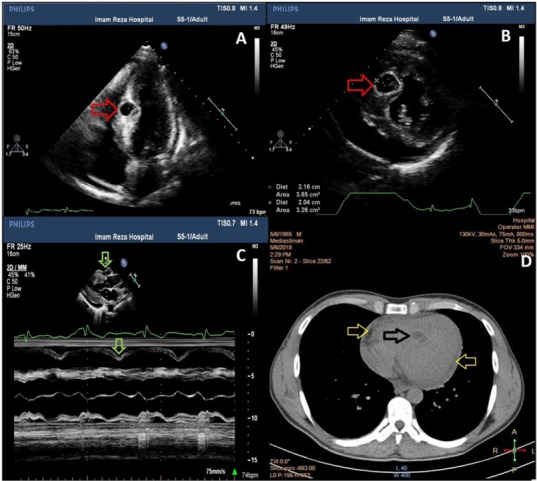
High-resolution computed tomography (HRCT) revealed a hypodense, cystic like structure in IVS and also pericardial effusion (Figure 2D). HRCT of the lungs and CT of abdominal area showed no additional organ involvement. Because of echocardiographic evidence of tamponade, increase in cTni and reduced LV ejection fraction, we consider perimyocarditis which can be related to hydatid cyst reactively. Therefore, surgery was planned for pericardiocentesis and excisional biopsy of cytic-like mass.
